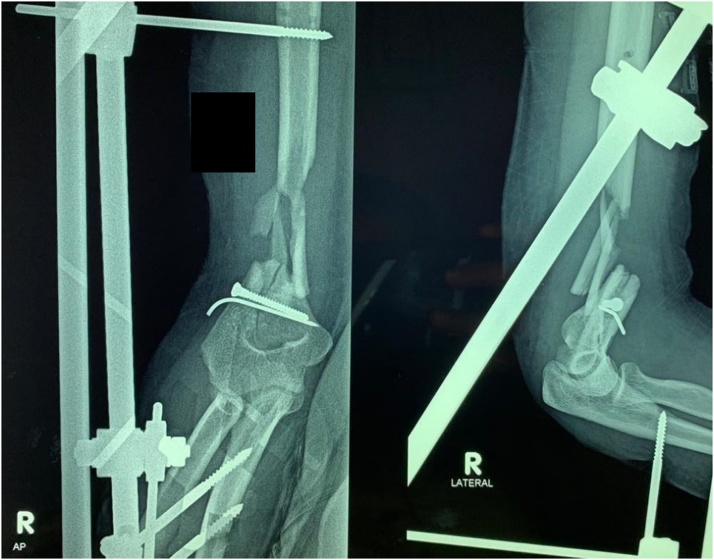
An x-ray made when the patient came to our hospital for the first time. The patient underwent debridement and external fixation in the previous hospital (source: internal documentation).
